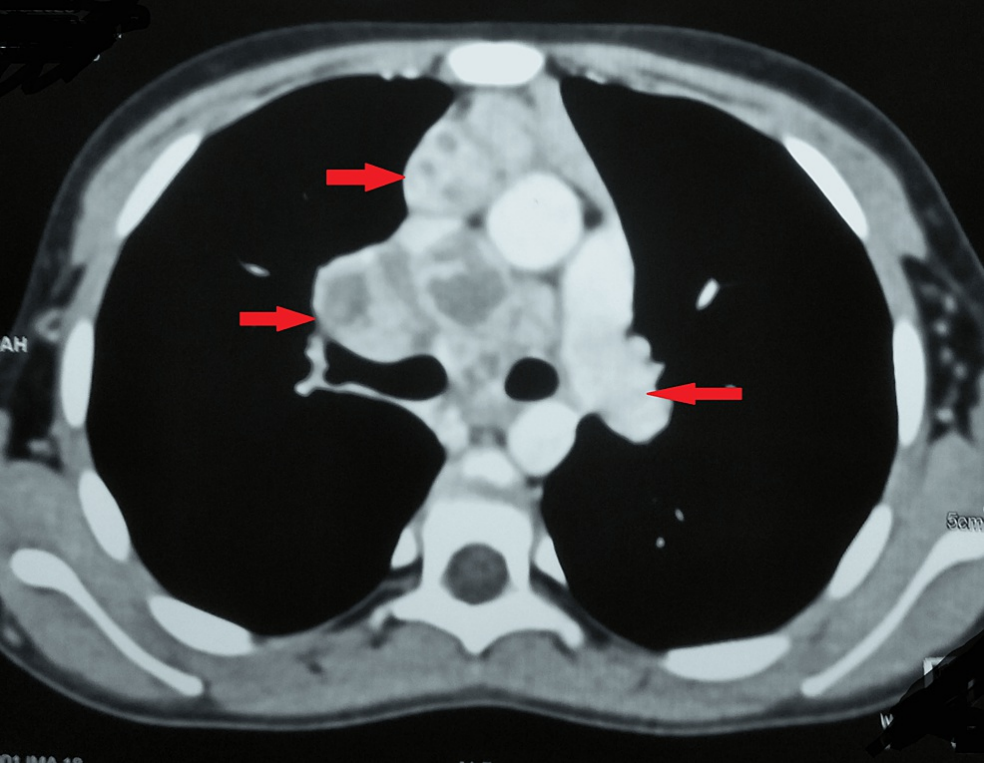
Computed tomography of the chest Arrows showing several enlarged conglomerated necrotic lymph nodes in the mediastinum
